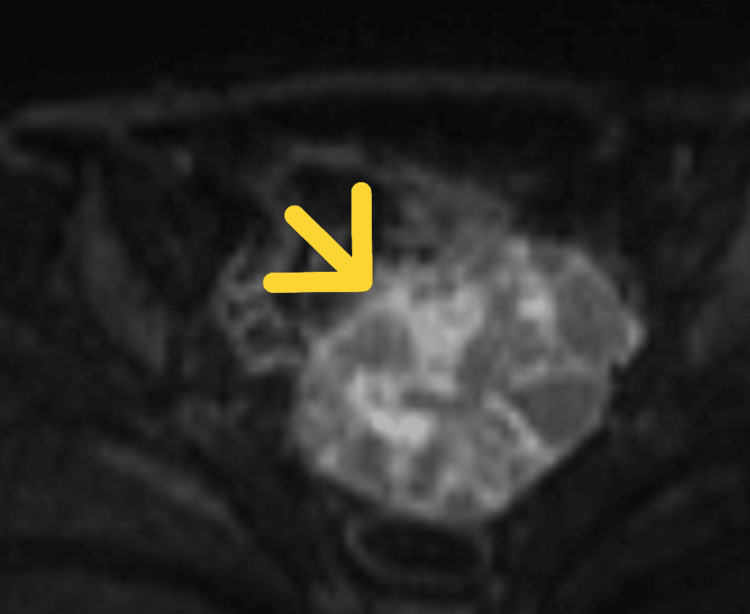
Diffusion-weighted MRI showing the ovarian mass Diffusion-weighted MRI showing the multilocular cystic lesion with focal areas of diffusion restriction, suggestive of high cellularity and supporting the suspicion of ovarian malignancy. The arrow indicates the ovarian mass.
